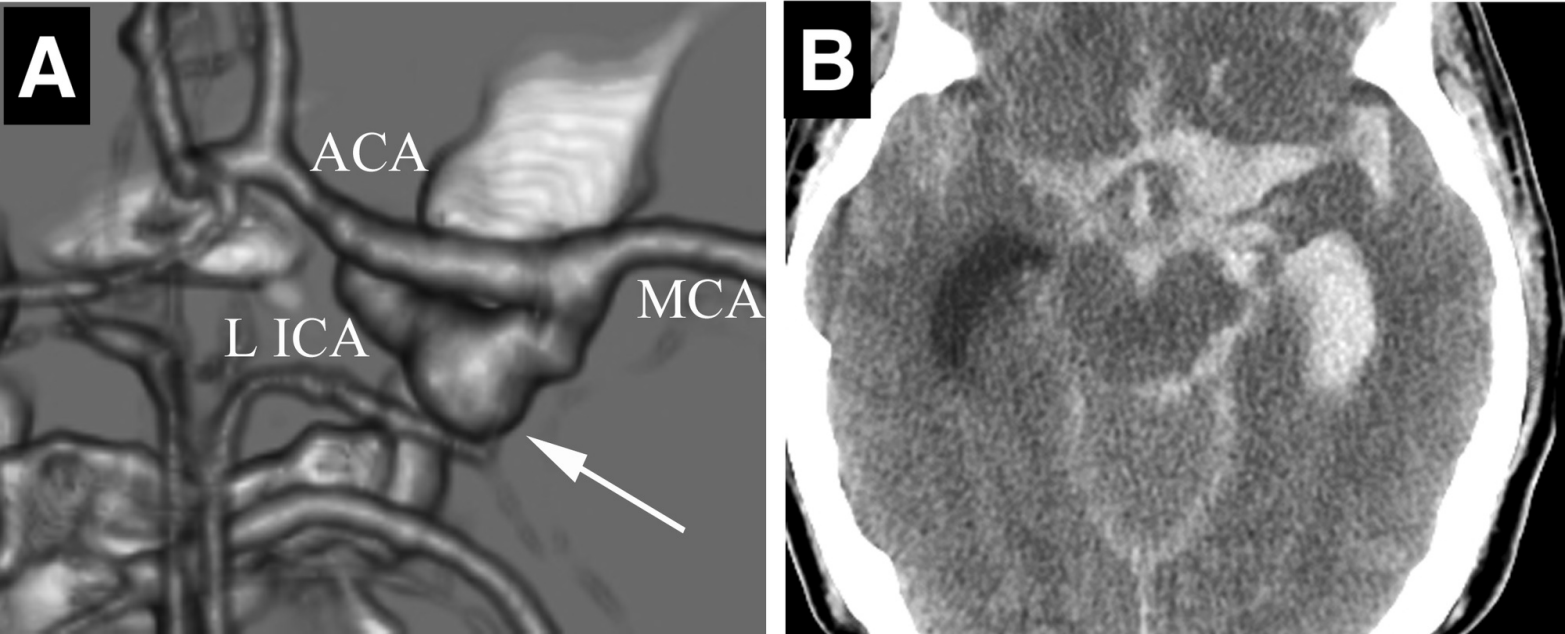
Repeat Imaging Studies A) 3Dimensional reconstruction of computed tomography (CT) angiography of the brain showing de novo blister aneurysm formation in the left supraclinoidal ICA (*arrow)*. B) Axial CT of the brain showing extensive subarachnoid hemorrhage from blister aneurysm rupture; *ACA- anterior cerebral artery, L ICA- left internal carotid artery, MCA- middle cerebral artery.*
